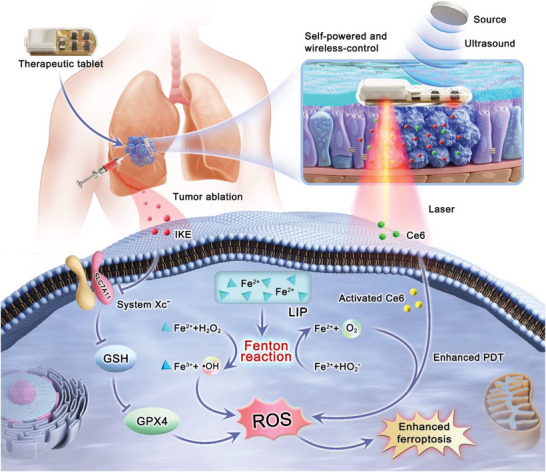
Ferroptosis inducer IKE combined with photodynamic therapy and underlying mechanisms for cancer treatment. Ferroptosis is induced in tumor cells by IKE via local inhibition of the SLC7A11‐GSH‐GPX4 signaling axis. In parallel, iron ions accumulate in the body, and via the Fenton reaction, improve the cellular hypoxic environment. The therapeutic tablet is minimally invasively implanted into the primary lung cancer in situ, and the µLEDs are driven to work by the action of ultrasound to activate the photosensitizer chlorin e6 (Ce6) using the oxygen supplemented by IKE for enhance photodynamic therapy, generate ROS, consume GSH. Lung cancer cells can be killed with ferroptosis because of the accumulation of ROS, which form lipid peroxides.
